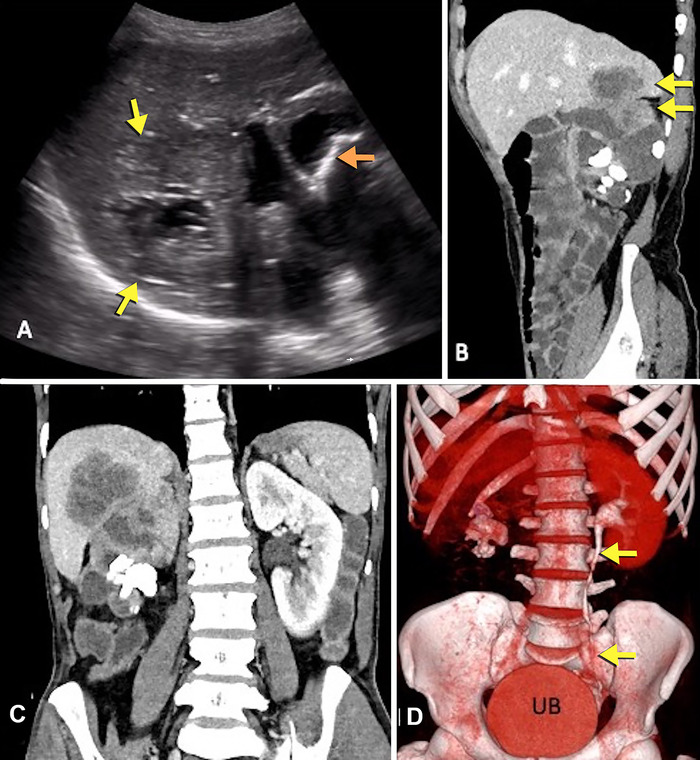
(A) Sagittal ultrasound image shows a heterogeneous mass in the upper pole of the right kidney with contiguous extension in segment VI of the liver and a central necrotic component (arrows on the left). Renal calculus with distal acoustic shadowing (arrow on the right) and hydronephrosis are also present. (B) Sagittal and (C) coronal reformats of contrast-enhanced computed tomography show multiple right staghorn renal calculi with hydronephrosis. A heterogeneously enhancing mass in the upper pole of the right kidney infiltrates the adjacent liver (arrows). (D) Excretory phase volume-rendered image shows right staghorn calculus with nonfunctioning right kidney. The left renal pelvis, ureter (arrows), and urinary bladder are delineated due to normal contrast excretion. UB, urinary bladder.
